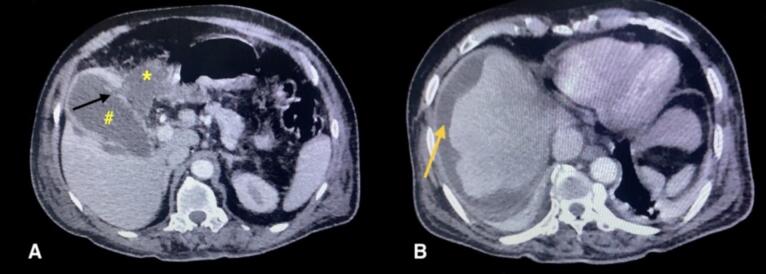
CECT abdomen axial section demonstrating Panel A: Gallbladder perforation at the fundus, indicated by a black arrow. A pericholecystic fluid collection is noted and marked with an asterisk (*). The gallbladder is marked with a hash symbol (#). Panel B: Perihepatic fluid collection is visualized and marked by a yellow arrow. CECT: Contrast-enhanced computed tomography. (For interpretation of the references to colour in this figure legend, the reader is referred to the web version of this article.)
